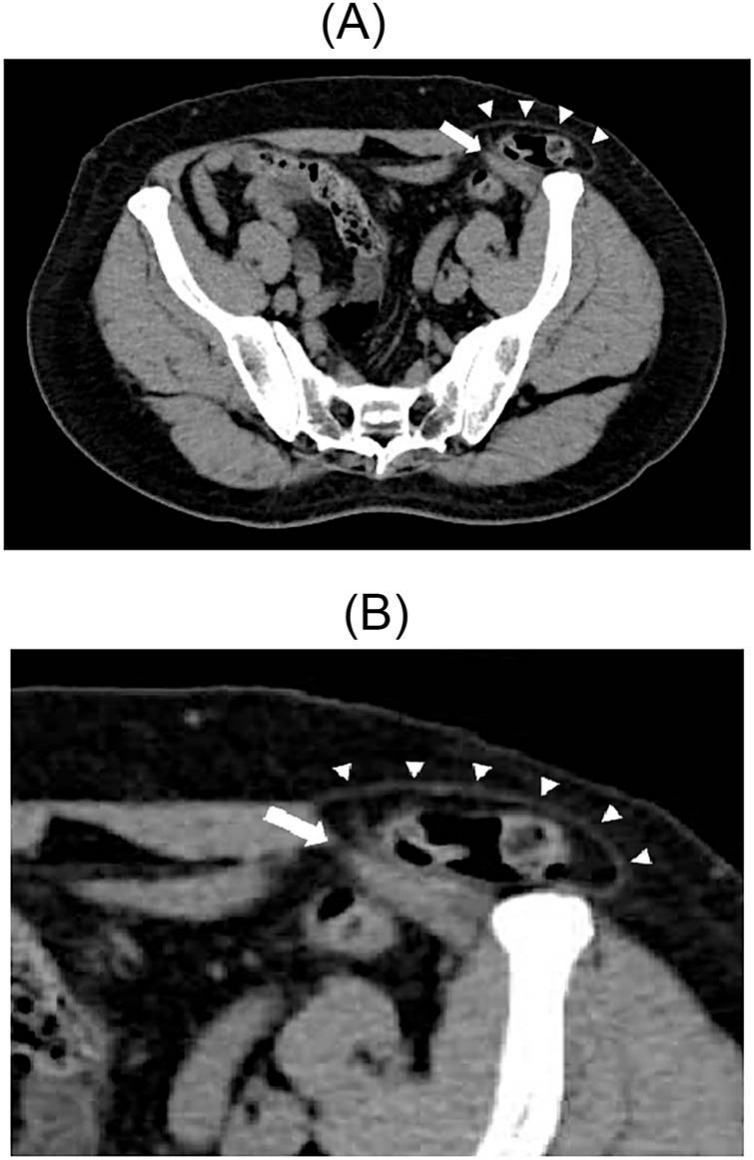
Contrast-enhanced CT scan; the sigmoid colon entered into the layer between the internal and external oblique muscles; the white arrow indicates the internal oblique muscle, and the white triangles indicate the external oblique muscle (A). Enlarged view of the inguinal region (B).
